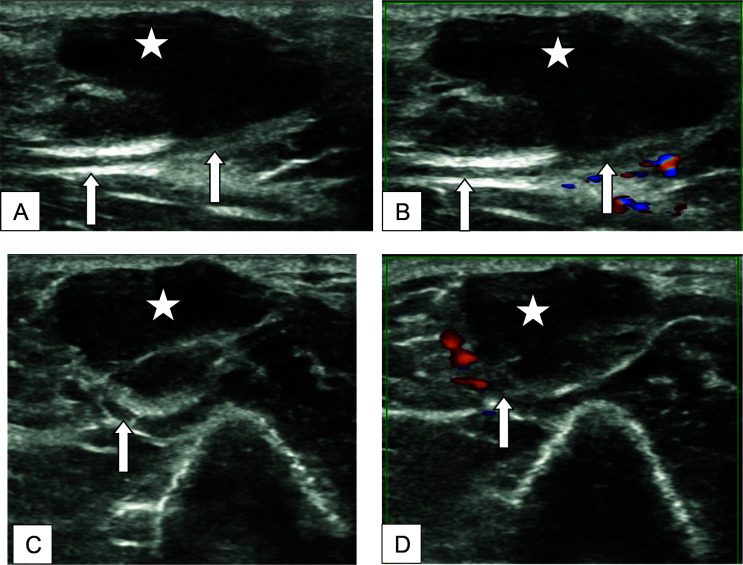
(A) Longitudinal gray scale, (B) Longitudinal color Doppler, (C) Axial gray scale, and (D) Axial color Doppler ultrasound images. Well-defined irregular-shaped hypoechoic lesion (star) with internal debris noted adjacent to the ulnar nerve (thick white arrows), not showing vascularity on color Doppler study.
